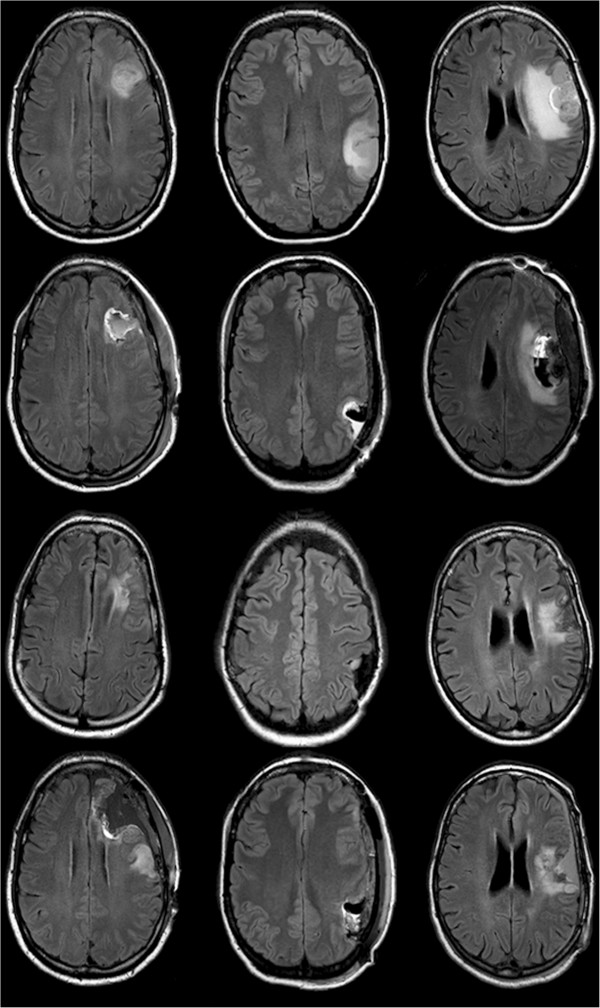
Pre- and postoperative MRI scans. Upper row: Initial tumor of patient 1–3; 2nd row: postoperative MRI of patient 1–3 shows resection after first surgery; 3rd row: recurrent tumor of patient 1–3; lower row: postoperative MRI of patient 1–3 shows resection after second surgery.
